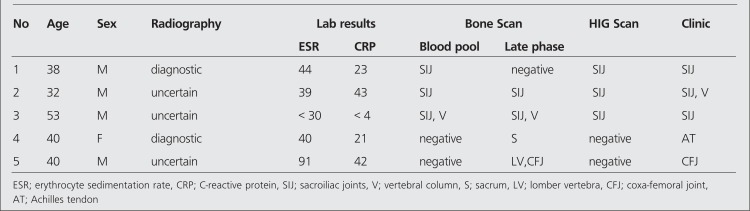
The demographic, radiographic and scintigraphic data of five patients who have clinically active disease
